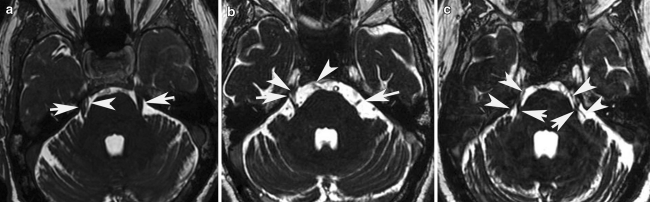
Axial MRI fast imaging employing steady-state acquisition (FIESTA) sequence showing superior cerebellar artery loop (*white arrow heads*) at root entry zone of trigeminal nerve (*white arrows*) on right side (a, b) and on both sides (c)
